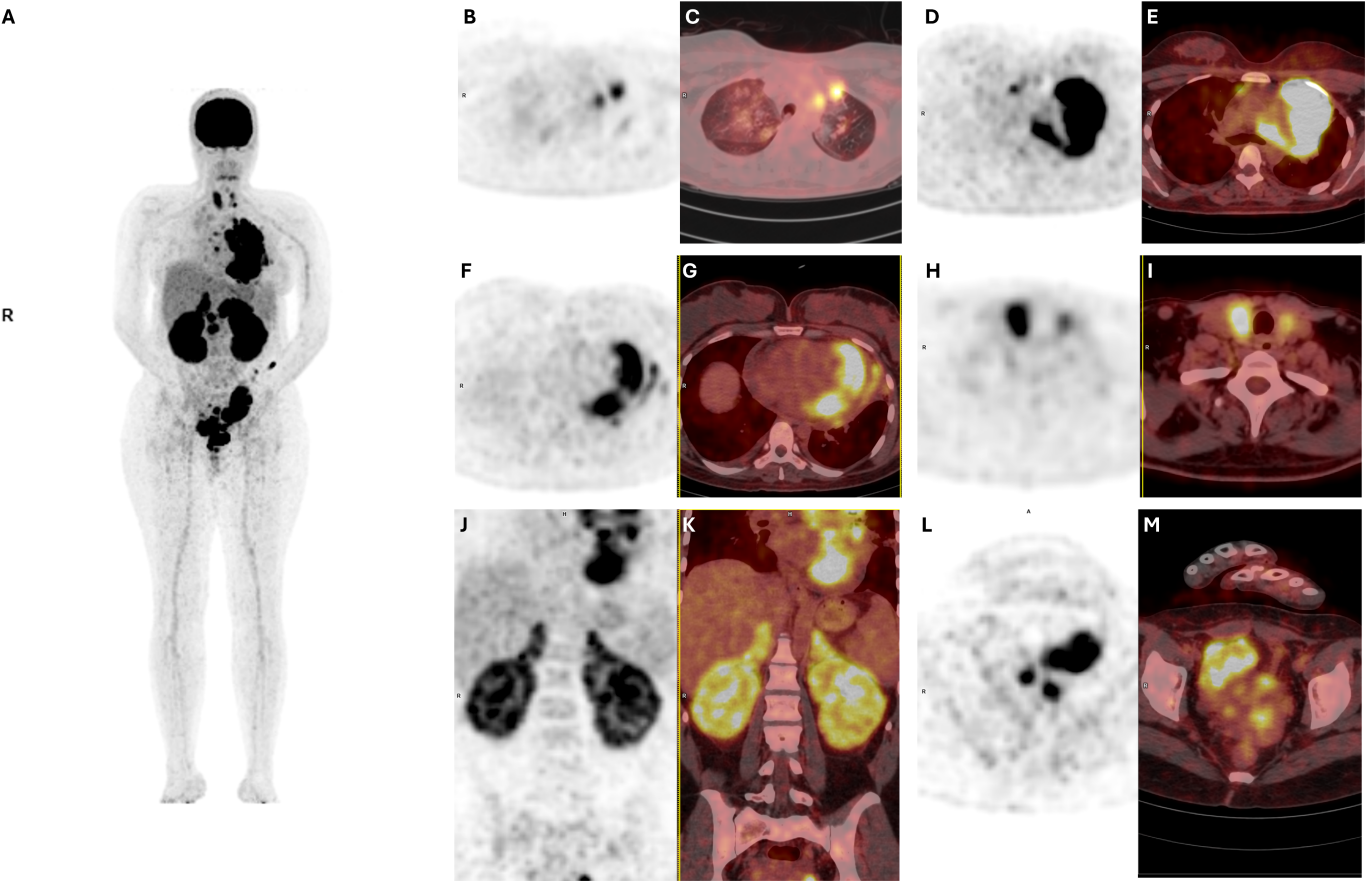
Baseline ^18F-FDG PET and fused PET/CT images obtained prior to treatment demonstrating extensive extranodal disease involvement. (A) Whole-body PET maximum intensity projection (MIP) demonstrating extensive metabolically active extranodal disease. (B, C) PET and corresponding fused PET/CT images demonstrating intense FDG uptake within bilateral pulmonary parenchymal lesions. (D, E) PET and corresponding fused PET/CT images showing FDG-avid supradiaphragmatic extranodal involvement. (F, G) PET and corresponding fused PET/CT images demonstrating marked FDG uptake involving the cardiac myocardium, consistent with myocardial infiltration. (H, I) PET and corresponding fused PET/CT images demonstrating diffuse thyroid gland hypermetabolism. (J, K) PET and corresponding fused PET/CT images demonstrating diffuse hypermetabolic involvement of the kidneys and adrenal glands. (L, M) PET and corresponding fused PET/CT images showing increased FDG uptake within the genitourinary organs.
